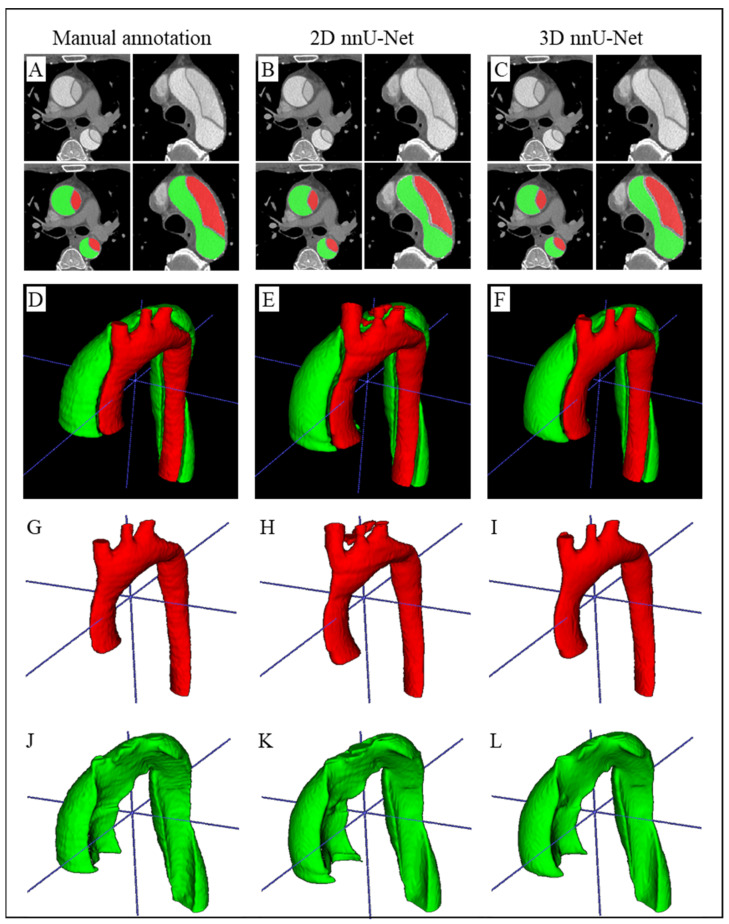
The 3D visualization of ascending aorta from manual annotation, 2D nnU-Net, and 3D nnU-Net. The four subgraphs in the first row (A–C) are two CT images of ascending aorta and arch and corresponding segmentation. (D–F) are the 3D visualization of ascending aorta from manual annotation, and prediction of 2D and 3D nnU-Net. (G–I) are the 3D visualization of TL. (J–L) are the 3D visualization of FL. Red region, TL; green region, FL.
